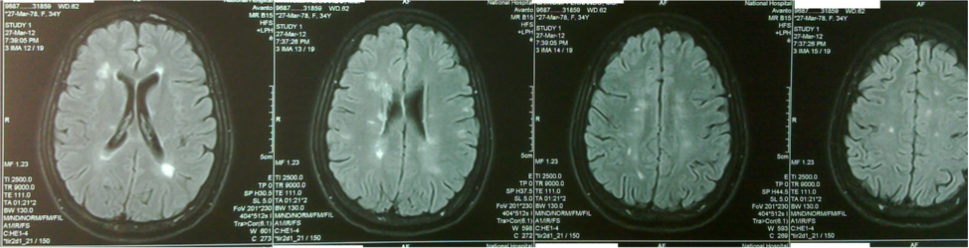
T2WI MRI brain images reveal multiple, hyperintense, periventricular deep white matter lesions highly suggestive of multiple sclerosis.
